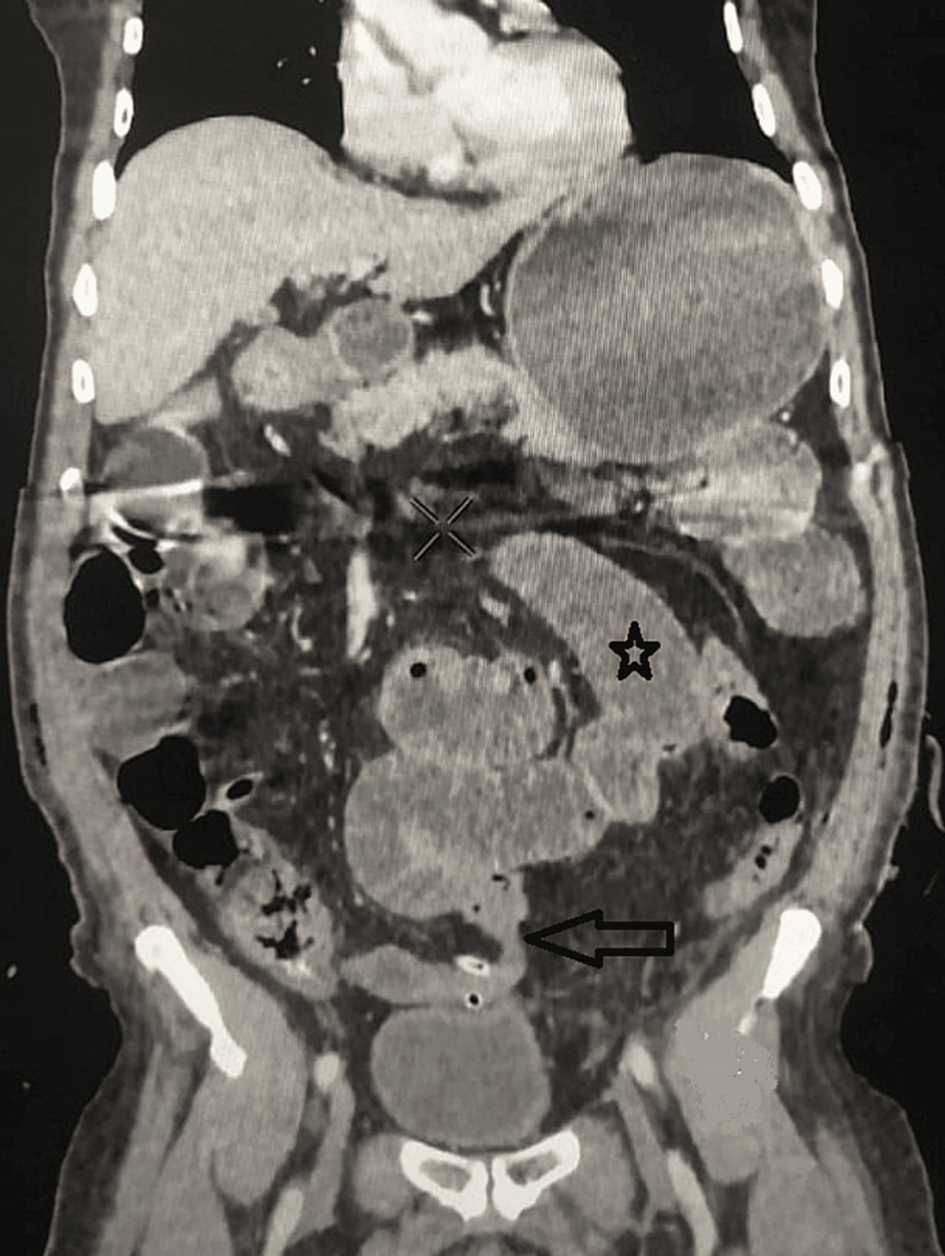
CT scan showing multiple segments of dilated small bowel measuring up to 4.7 cm (asterisk) with a transition point (arrow) related to an obstruction at the surgical drain site.
